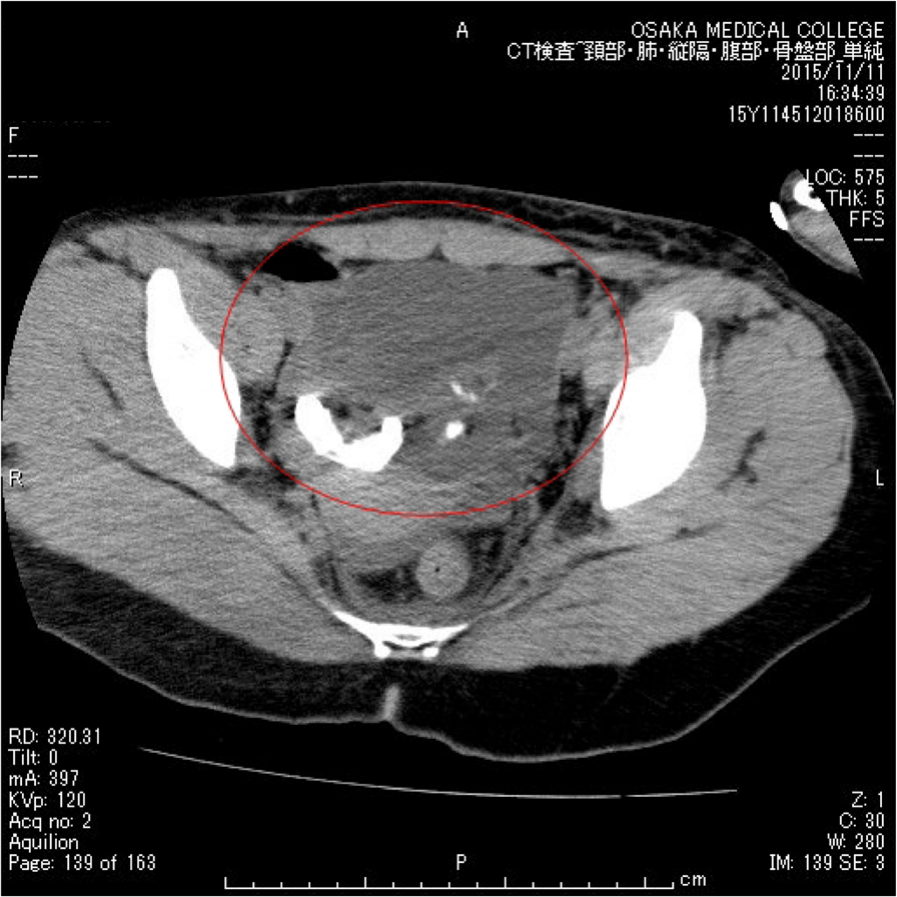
Abdominal CT image showing a right cystic adnexal mass with an internal focus of fat and high-attenuation material, suggesting an ovarian teratoma (*red circle*)
